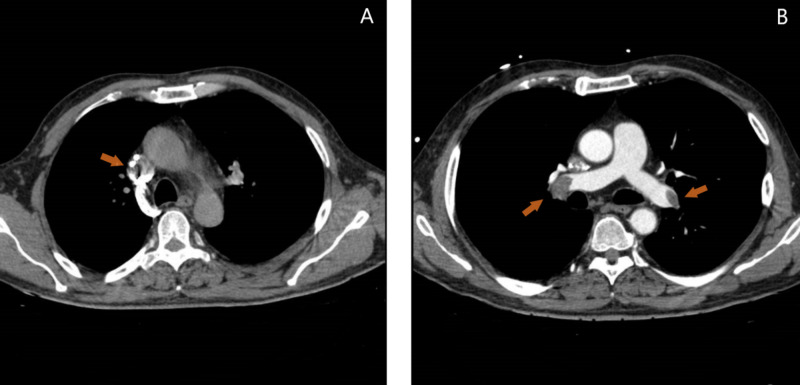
CTA findings. A: Computed tomography angiography (CTA) scan of the thorax with a nonocclusive thrombus in the superior vena cava (SVC) (arrow). B: Evidence of bilateral pulmonary thromboembolism (arrows).
